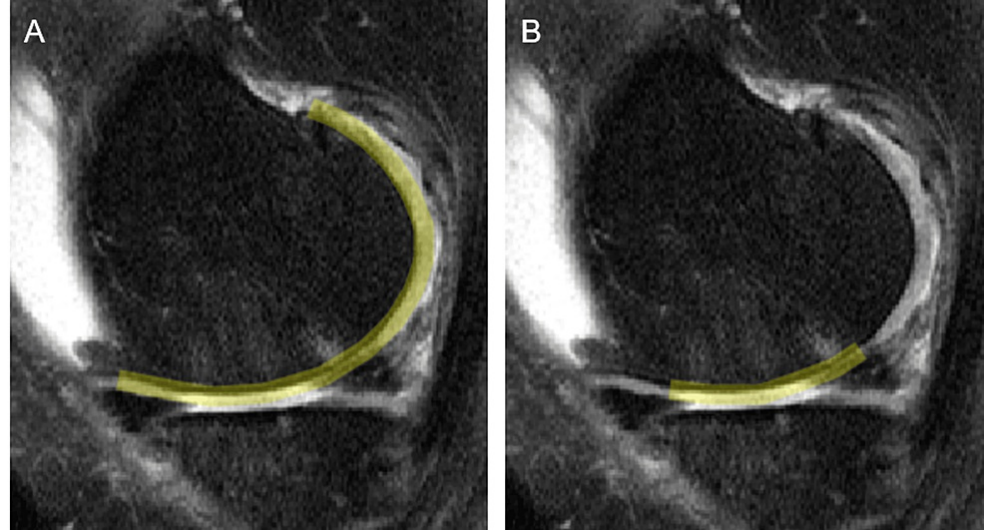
Example of cartilage loss fraction measurement on MRI. Sagittal IW FS sequence was used for the segmentation.  For each 2D image, completely denuded articular surface measurement (B, marked yellow) was divided by the subchondral bone measurement (A, marked yellow) and the full-thickness cartilage loss fraction was obtained. IW FS: intermediate-weighted fat saturation; 2D: two-dimensional
